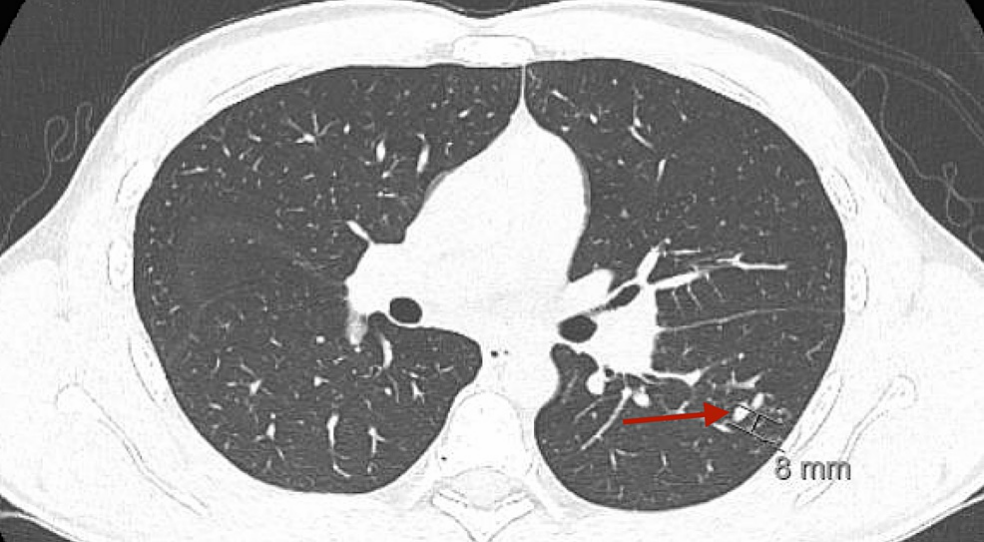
CT Thorax without contrast: elongated nodular lesion in the apical segment of the left lower lobe.
